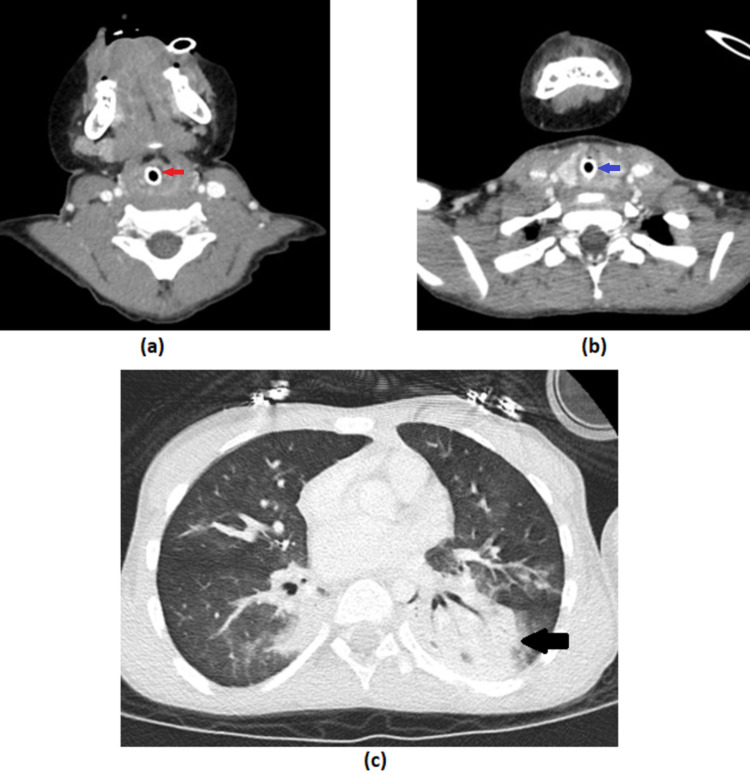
Computed tomography Axial plane CT scan with contrast whilst the patient was intubated. Images (a) and (b) demonstrate midline larynx (red arrow) and trachea (blue arrow) and absence of lesions, masses or vascular abnormalities in the neck. Image (c) demonstrates aspiration with collapse/consolidation of the lower lobes (left > right) (black arrow).
